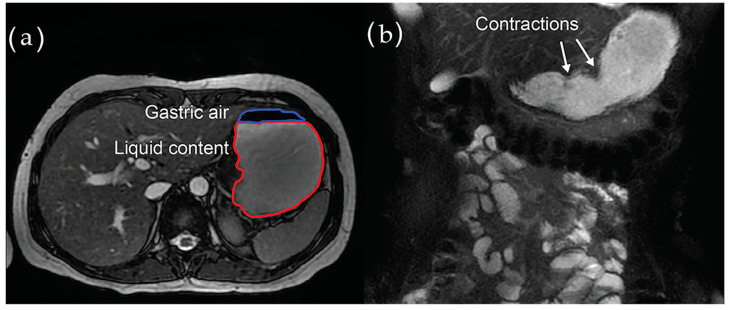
Magnetic resonance imaging of the stomach. (a) Gastric air volume and liquid content volume obtained in the segmentation process. (b) Contraction waves observed and quantified in the coronal plane.
